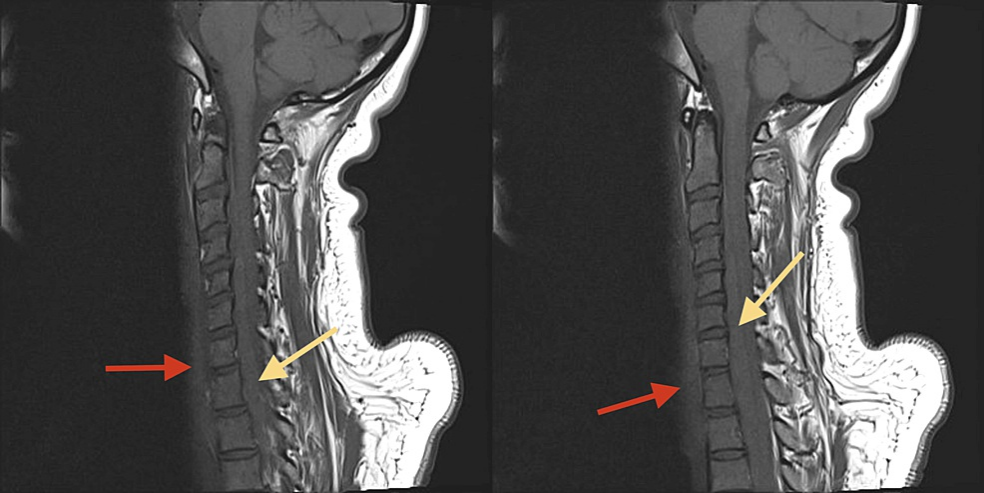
MRI SAG T1 WI reveals slightly hyperintense prevertebral (red arrow) and intraspinal haematoma (yellow arrow). SAG: Sagittal
